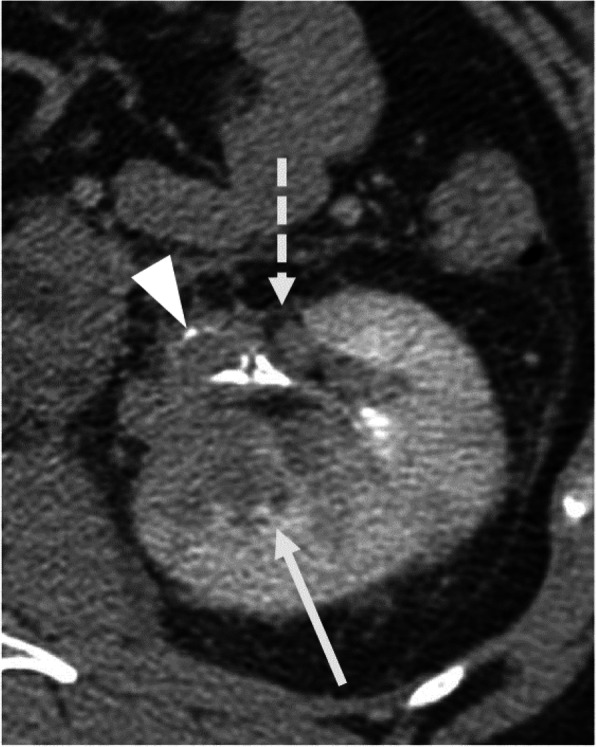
45-year-old female with metastatic fumarate hydratase-deficient renal cell carcinoma. Delayed phase post-contrast axial computed tomography showing endophytic renal mass with infiltrative margin (arrow), renal sinus fat invasion (dashed arrow), and hilar collecting system invasion (arrowhead)
